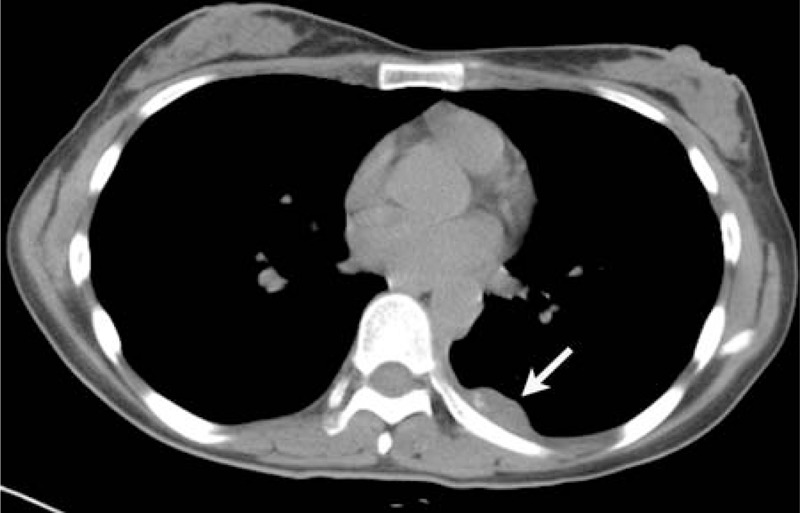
A 22-year-old woman presented with a history of left back pain for about 1 month. Unenhanced CT scan shows that the tumor had the amorphous calcifications in the posterior left chest wall (arrow).
